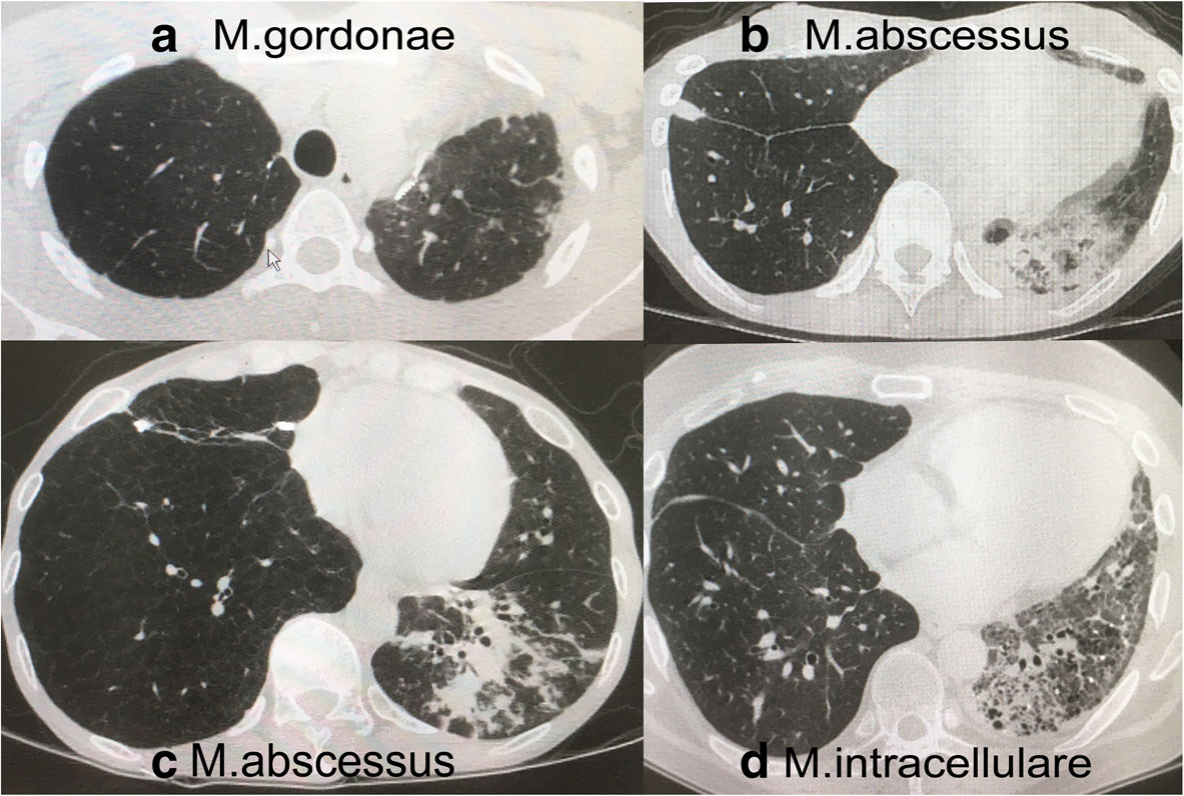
a–d. Chest computed tomography showing consolidation. a Case 1, slight consolidation in left upper lesion caused by *M. gordonae*. b Case 2, consolidation in left lower lesion caused by *M. abscessus*. c Case 3, consolidation in transplanted lung caused by *M. abscessus*. d Case 4, consolidation in native lung caused by *M. intracellulare*
